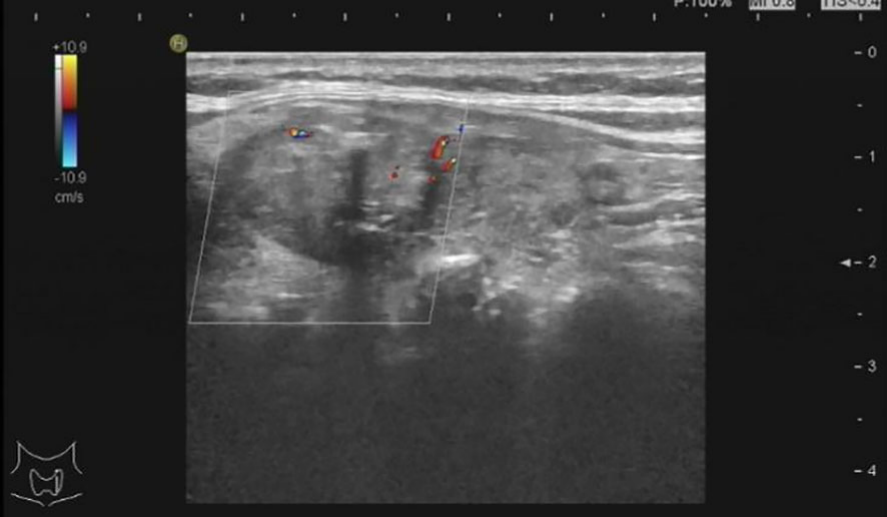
Thyroid color ultrasound image.
